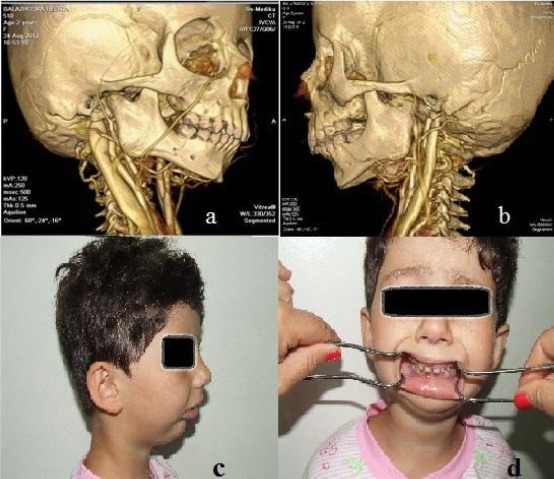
Preoperative three-dimensional CT: a. Right lateral view: the enlargement of the coronoid process protruding under inferior rim of the zygomatic arch; b. Left lateral view: the enlargement of the coronoid process protruding under the inferior rim of the zygomatic arch; c.d. lateral and frontal preoperative photograph (mouth opening prior to operation)*.
